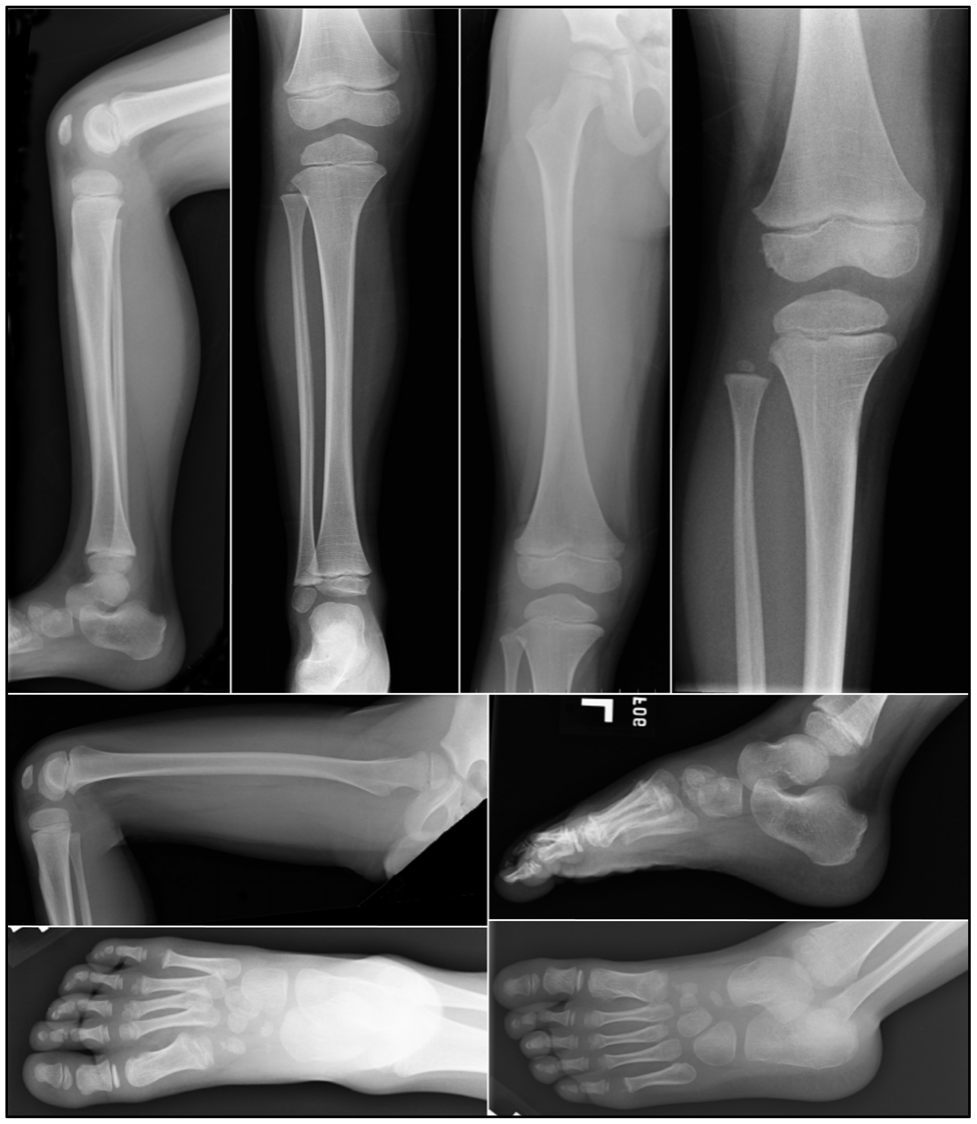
Radiographs of the proband’s left (dominant) leg at age 6. Radiographs show the femur, tibia, fibula, knee, and foot. No significant osteopenia, bony destructive process, periosteal reactions, or evidence of any acute fractures, dislocations, injuries, or remote traumatic changes were observed.
